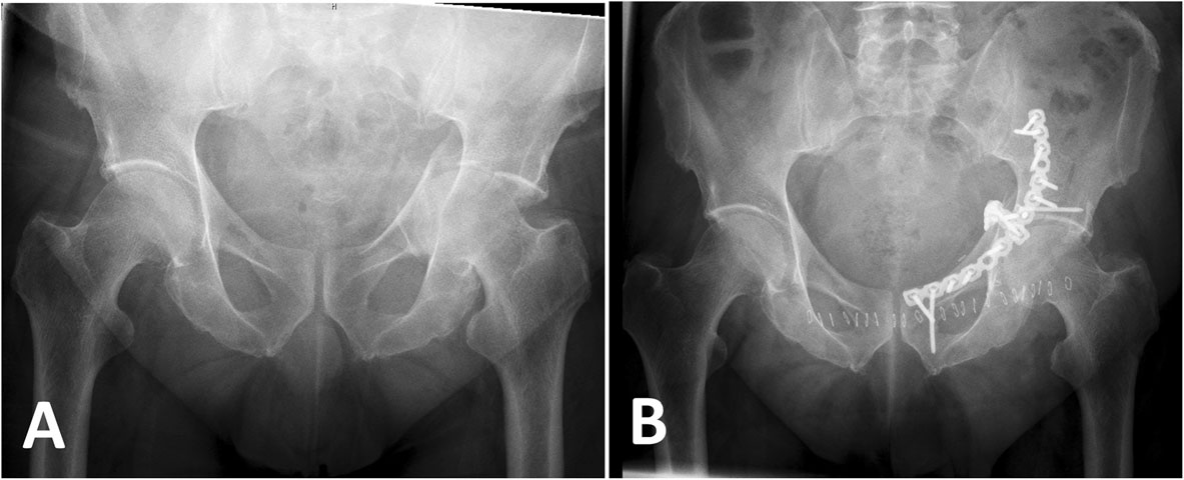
Case. Seventy-five year-old male patient with a left acetabulum fracture after a fall from standing height. a Pelvis AP radiograph on admission showing the central dislocation of the femoral head into the lesser pelvis. b Pelvis AP radiograph after open reduction and internal plate fixation
